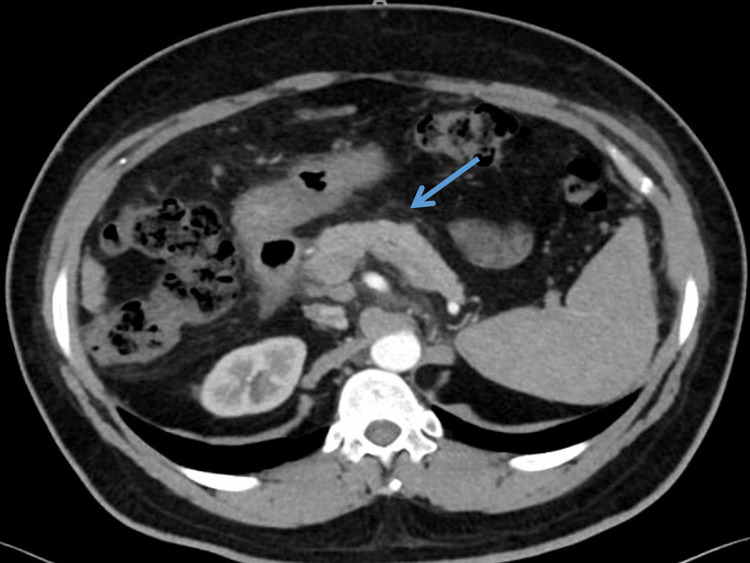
Contrast-enhanced computed tomography (CECT) abdomen showing normal pancreas (blue arrow) at six-month follow-up.
